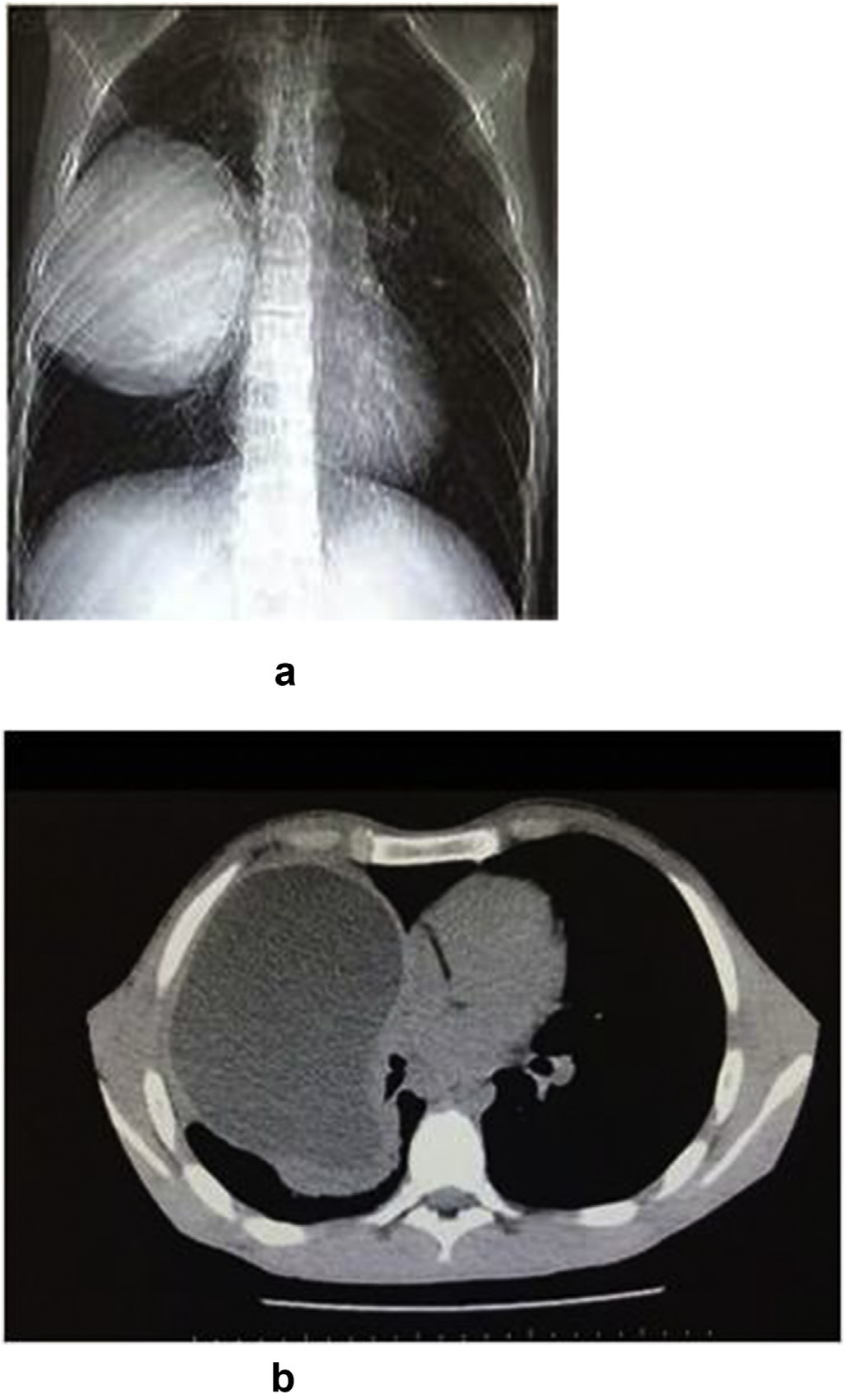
(A) Preoperative view of the Chest X-Ray. (B) Preoperative view of Thorax CT.
